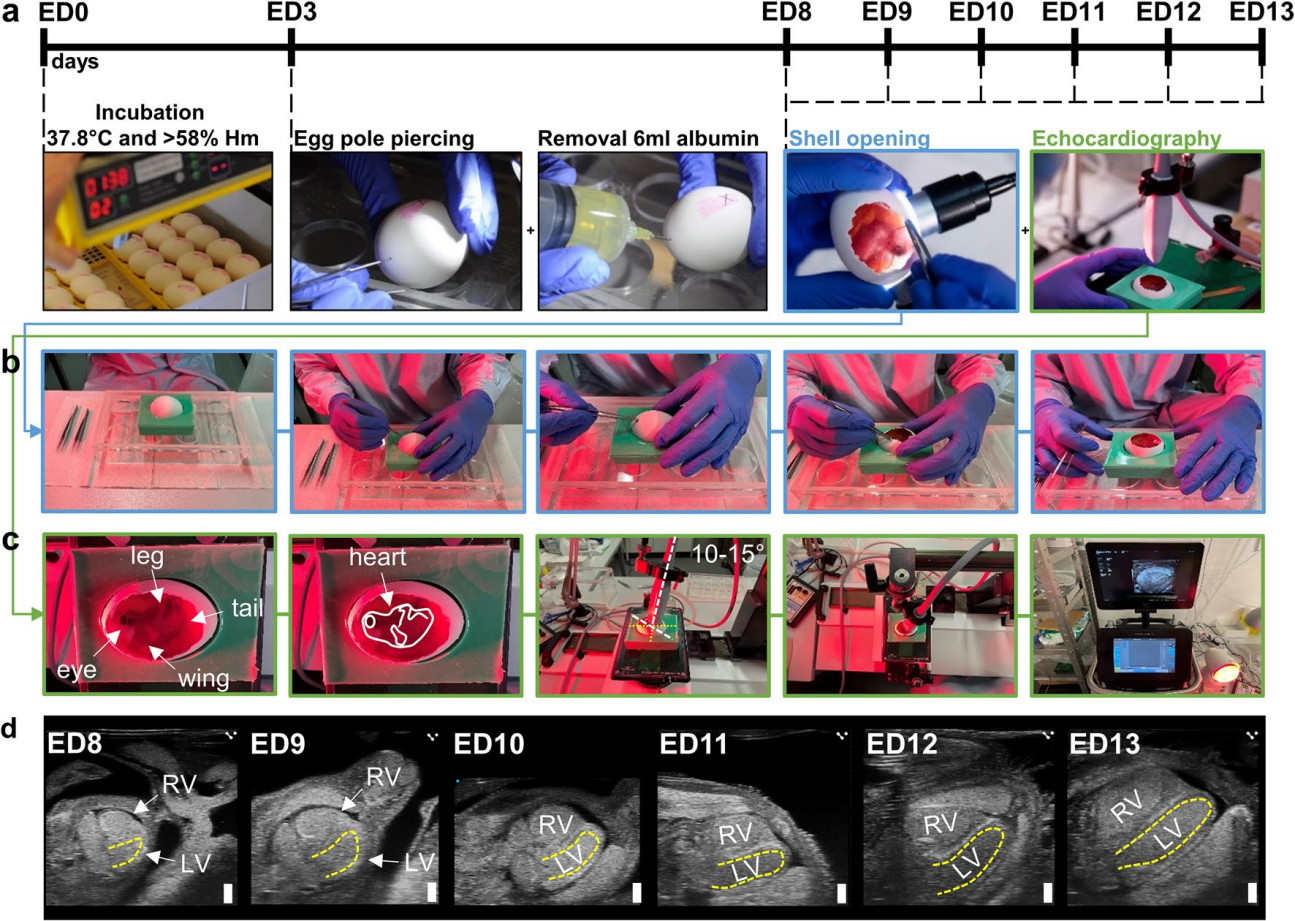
iCE incubation scheme and experimental outline. a iCEs were incubated starting ED 0 in a special incubator at 37.8 °C and > 58% humidity (Hm). On ED 3, the egg poles were pierced, and 6 ml of albumin were removed to lower the chorioallantoic membrane. b, c On ED 8 to ED 13 iCEs were subject to transthoracic echocardiography *in-ovo*. d Representative echocardiographic B-Mode images in the modified five-chamber view (^mod^5CV) of iCEs on ED 8 to ED 13. Yellow contours depict left-ventricular endocardial borders. *RV*  right ventricle, *LV*  left ventricle. Scale bar = 1 mm
